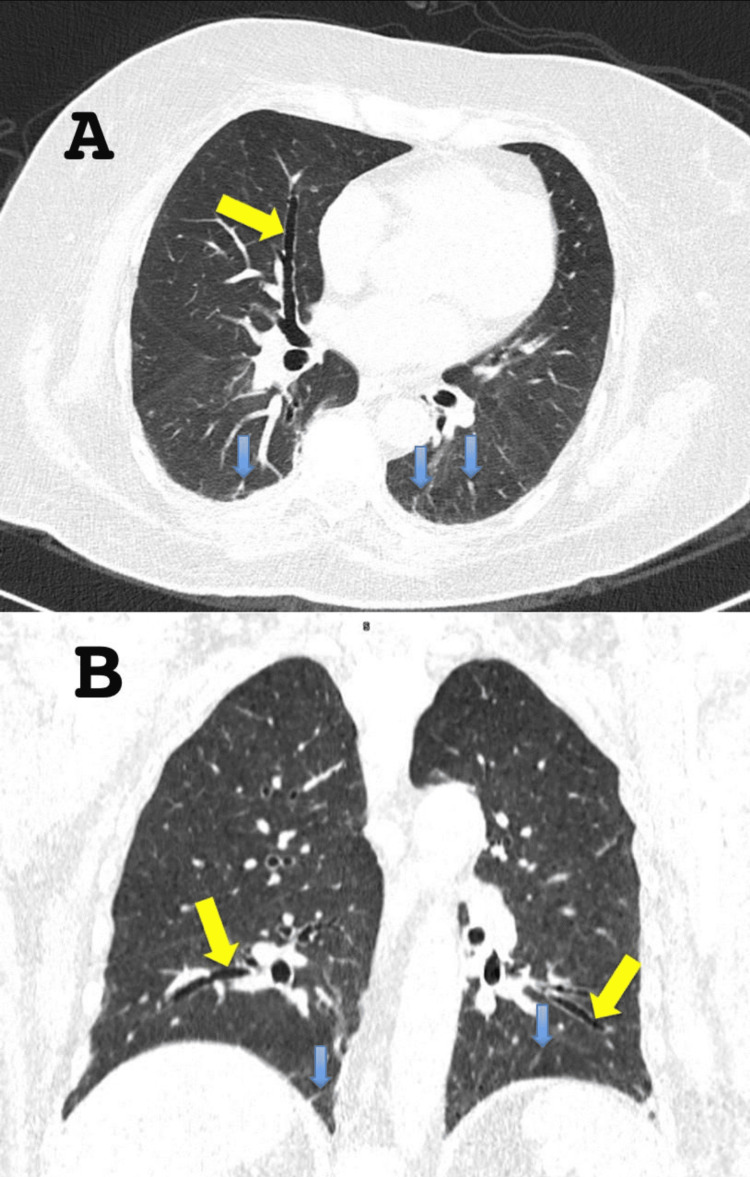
Computed tomography (CT) of the chest in lung window settings (A) Axial view demonstrates interstitial thickening (blue arrow) and cylindrical bronchiectasis (yellow arrow). (B) Coronal view confirms the presence of bilateral interstitial thickening (blue arrow) and cylindrical bronchiectasis (yellow arrow), predominantly in the lower lung zones. These findings are consistent with ILD. ILD: Interstitial lung disease
